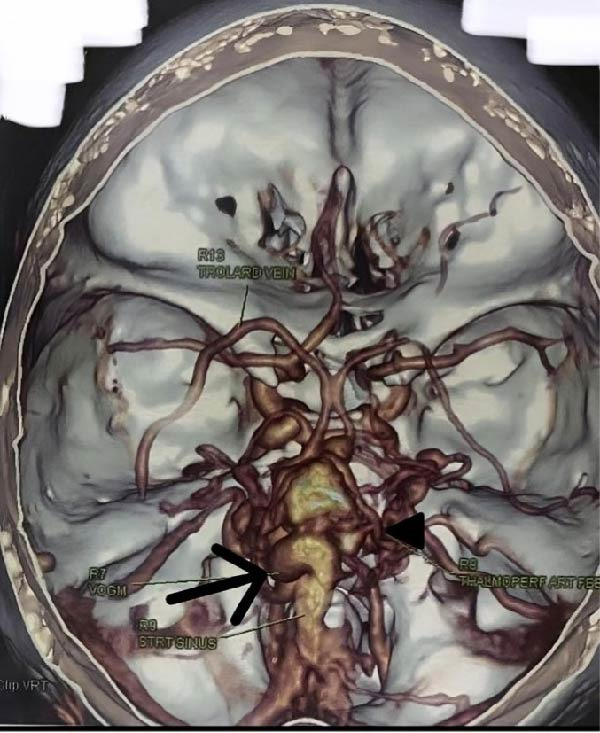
3‐Dimensional computed tomography (CT)—cerebral angiogram: aneurysmal dilatation and tortuosity of VoG with arterial feeders from the thalamoperforating and posterior‐choroidal arteries.
